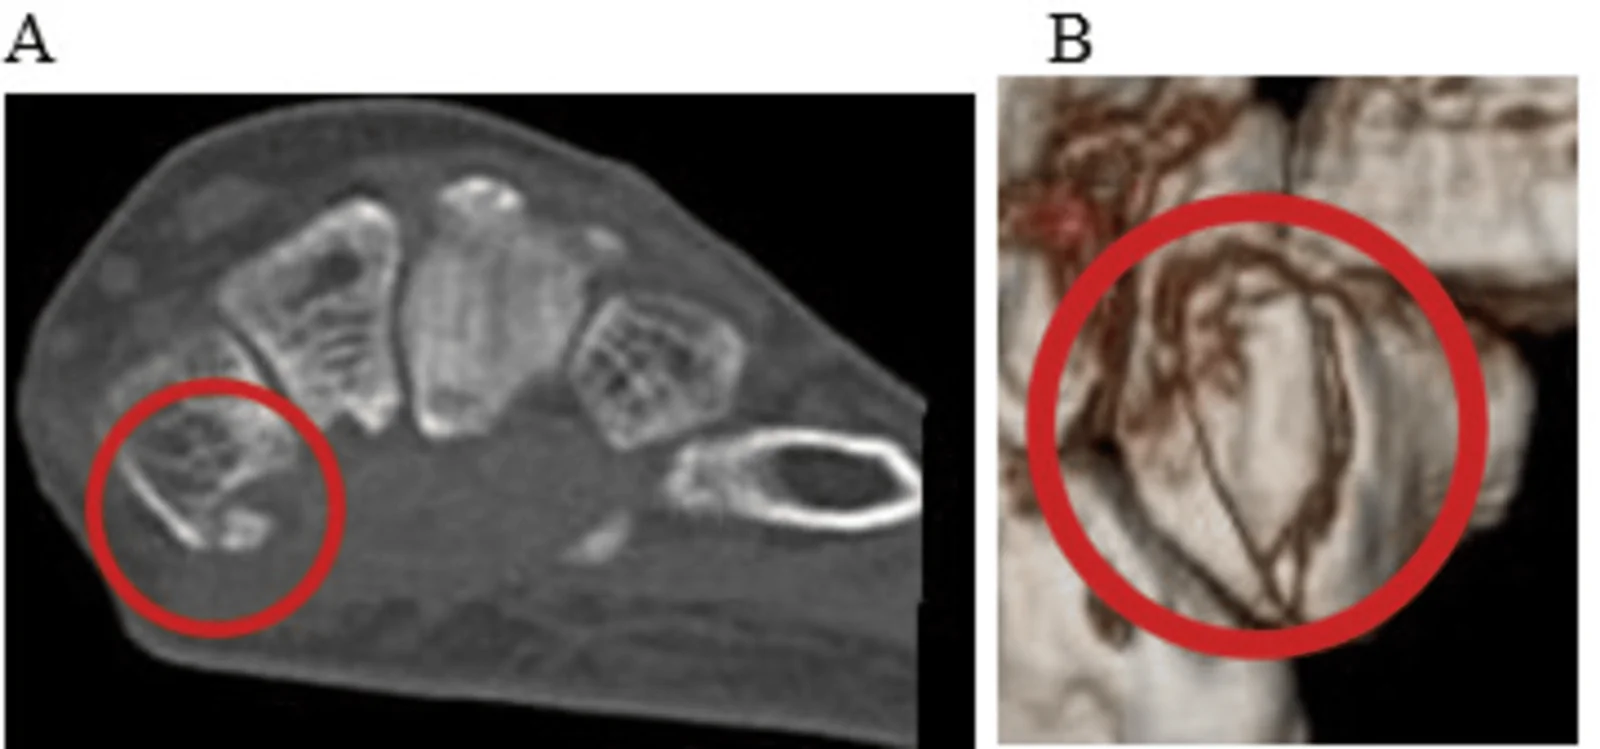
Case 2: CT images obtained four weeks post-injury. Axial (A) and three-dimensional (B) CT images showing an absence of both fracture exacerbation and callus formation (red circles).
